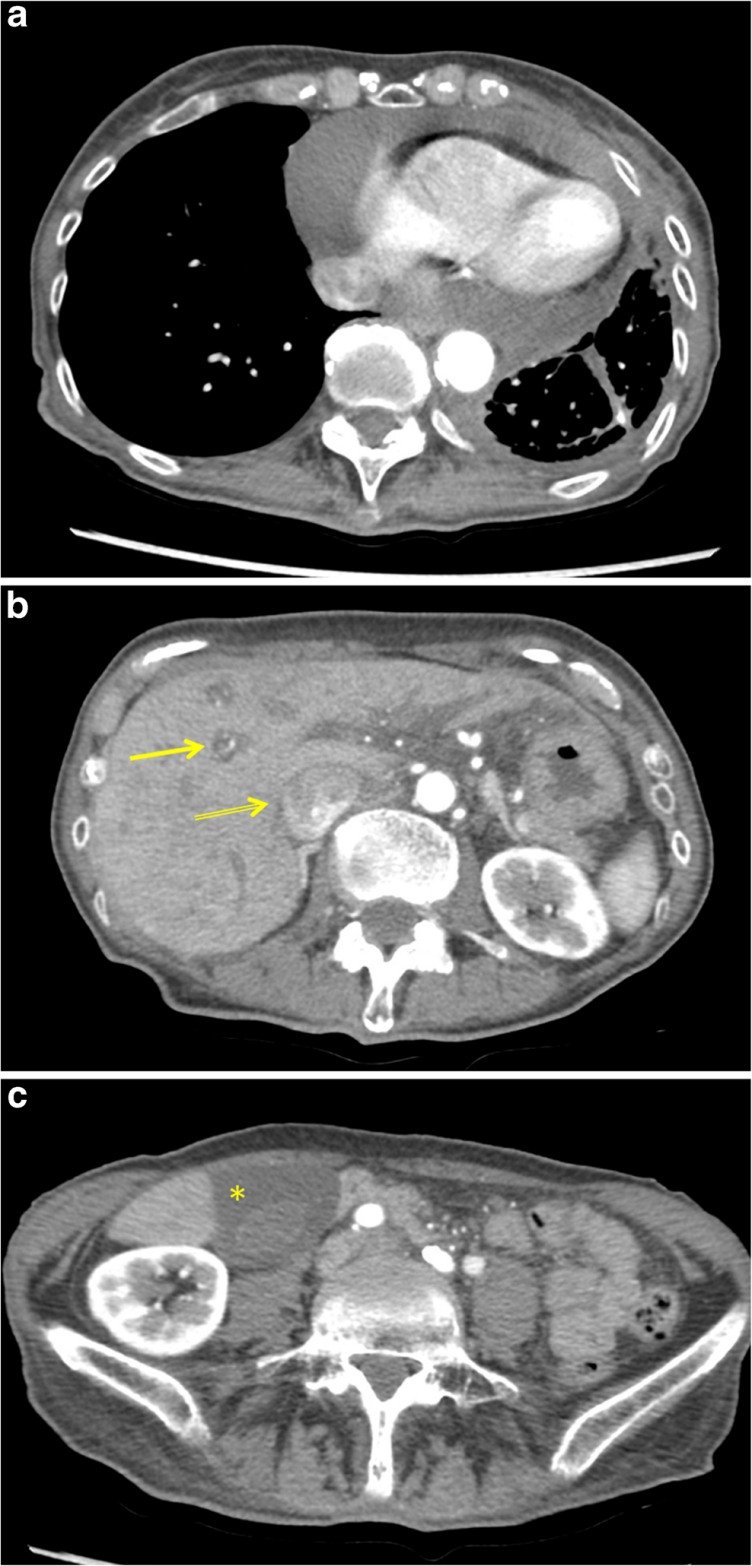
A 73-year-old woman with a known stage IV non-small cell lung cancer presented during follow-up with symptoms of chest pain, increasing dyspnea and fatigue. a Contrast-enhanced axial CT image in mediastinal window setting depicts a massive pericardial effusion without prominent pericardial thickening, nodularities or enhancement. b, c Axial CT images at the level of the upper abdomen show an enlargement of the inferior vena cava with contrast reflux (*double yellow arrow*), periportal oedema (*yellow arrow*) and gallbladder oedema (*yellow asterisk*) as signs of right heart failure. Findings are compatible with cardiac tamponade. The woman was referred for urgent cardiac work-up and drainage of the cardiac fluid
